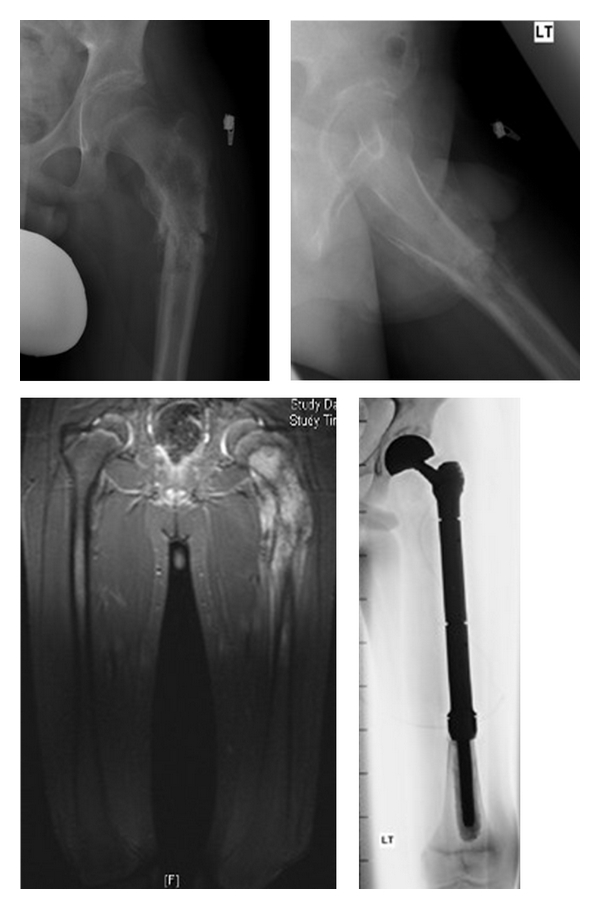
Preoperative radiographs, MRI scan of osteosarcoma of the proximal femur, and postoperative radiograph showing proximal femoral endoprosthesis.
